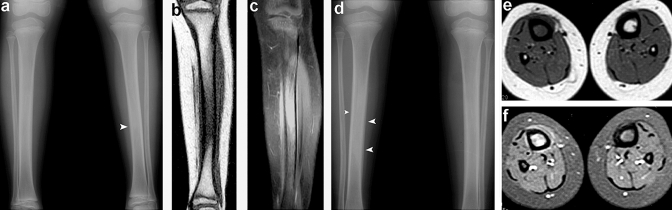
(a) Radiograph demonstrating a subtle periosteal reaction in the midshaft of the left tibia (arrowhead). (b) A coronal T1-weighted sequence showing loss of normal fatty marrow in the tibia. (c) A post-contrast fat-suppressed coronal T1-weighted image revealing contrast enhancement in the marrow and adjacent soft tissues. (d) Radiograph showing a periosteal reaction (arrowheads) and medullary sclerosis of the right tibia. (e) An axial T1-weighted image demonstrating reduced bone marrow signal of the right tibia with adjacent soft tissue intensity. (f) A post-contrast fat-suppressed axial T1-weighted image revealing contrast enhancement in the marrow and juxtacortical soft tissue enhancement in the right tibia.
